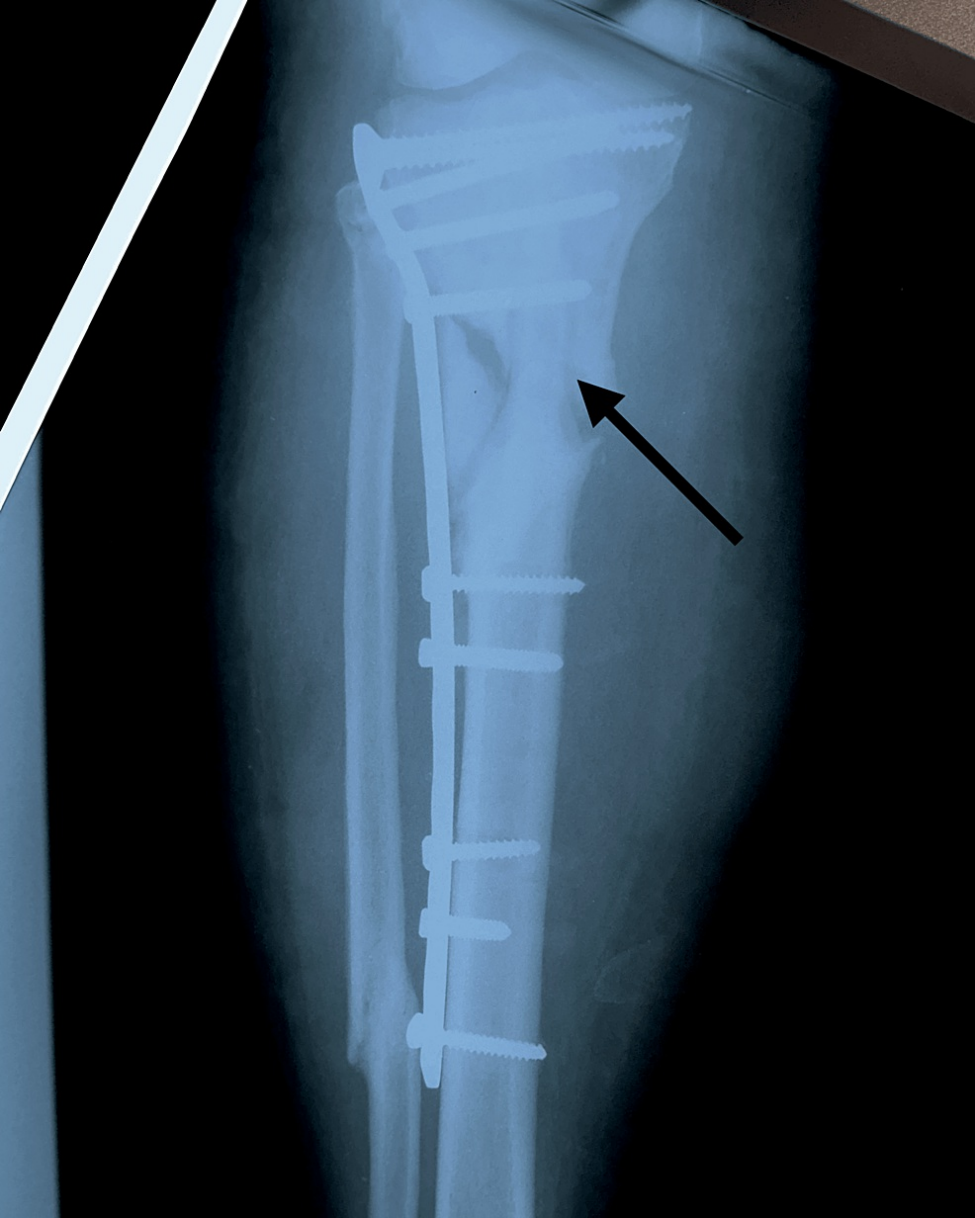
Postoperative anteroposterior X-ray of right leg showing fibular strut graft, proximal tibia locking plate and screws (arrow points to the fibular strut autograft in the tibia medullary canal)
